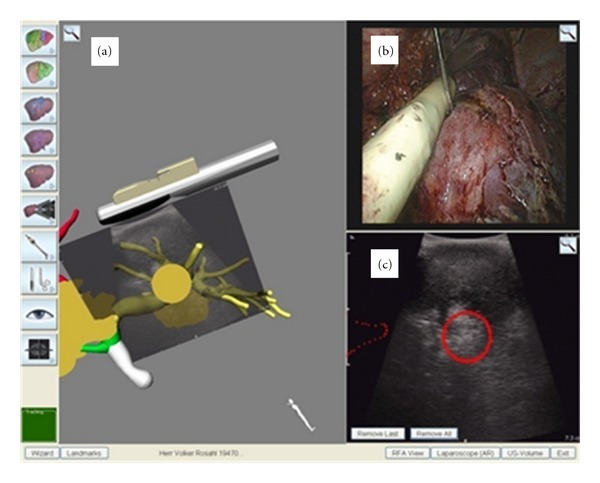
(a–c) Screen shot of the LapAssistent with simultaneous presentation of (a) virtual reality navigation with registered intraoperative B-Mode ultrasound picture to the preoperative 3D-MRI-planning data, (b) real-time laparoscopic video screen imported from surgical laparoscopic imaging device, and (c) real-time ultrasound B-Mode picture with overlapping tumor.
